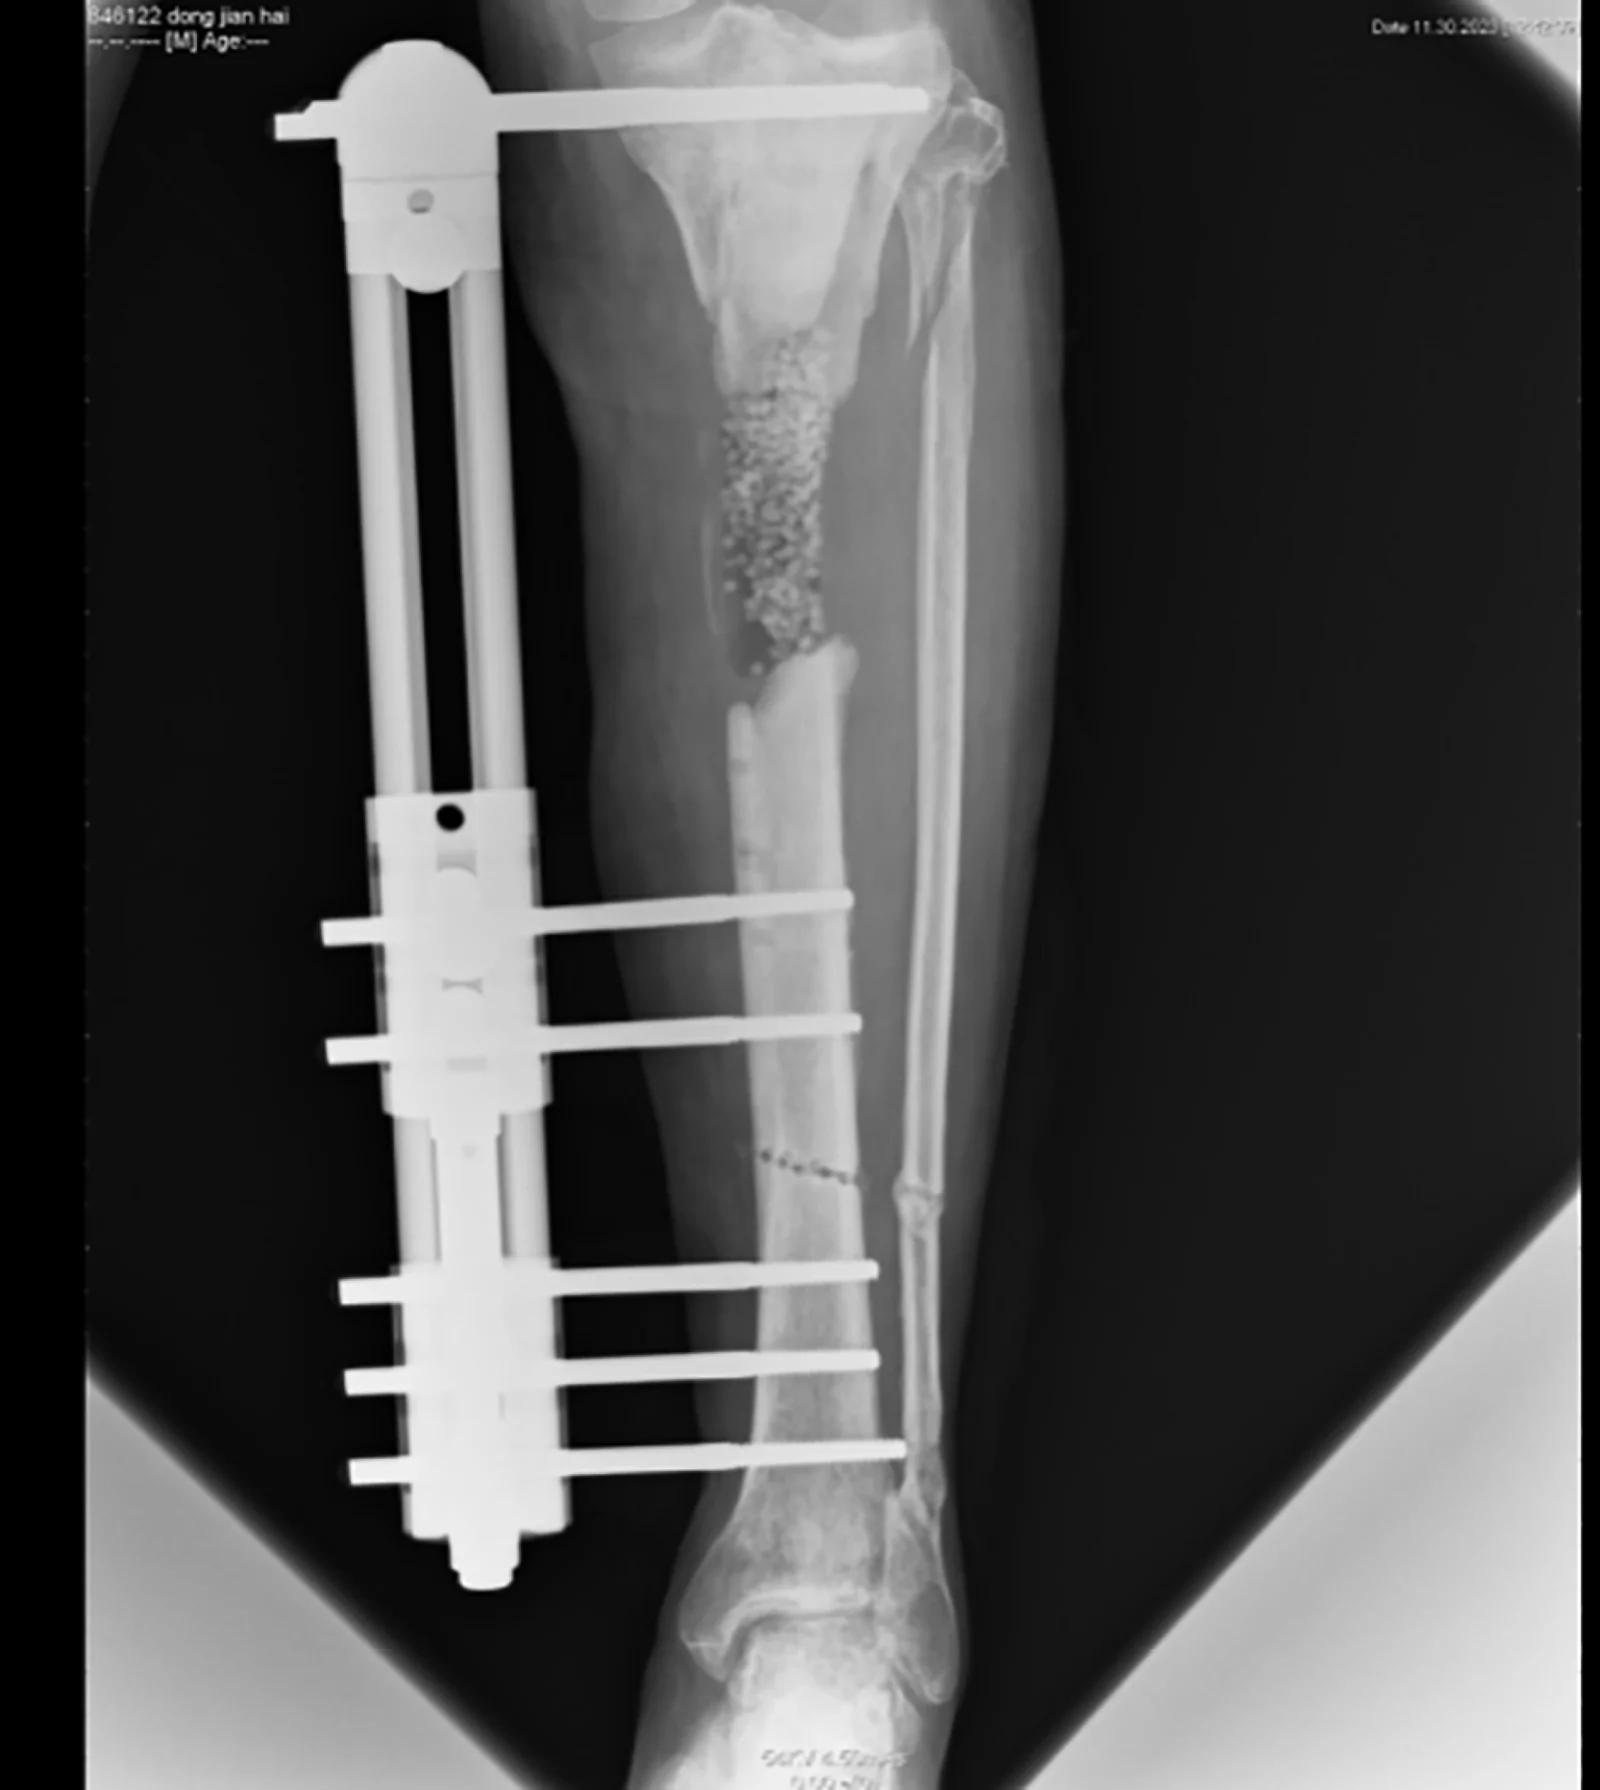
Radiographic appearance after the second-stage surgery. Following the removal of the ALBC spacer, a distal tibial osteotomy has been performed. The bone transport segment has been mobilized, initiating the distraction osteogenesis process to gradually close the bone defect.
